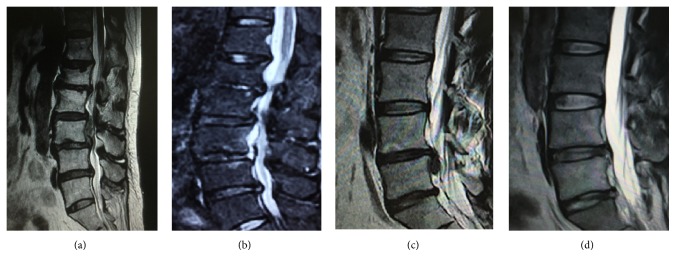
Preoperative and postoperative imaging examination. (a) Preoperative magnetic resonance imaging (MRI) revealed L4/5 disc prolapse with nucleus shifting upward to L3/4 intervertebral space. (b) Postoperative MRI examination revealed clean removal of the nucleus pulposus, with no compression of the L4/5 nerve root. (c) Preoperative magnetic resonance imaging (MRI) revealed L4/5 disc prolapse with nucleus shifting downward to L5 vertebral posterior. (d) Postoperative MRI examination revealed clean removal of the nucleus pulposus, with no compression of the L4/5 nerve root.
